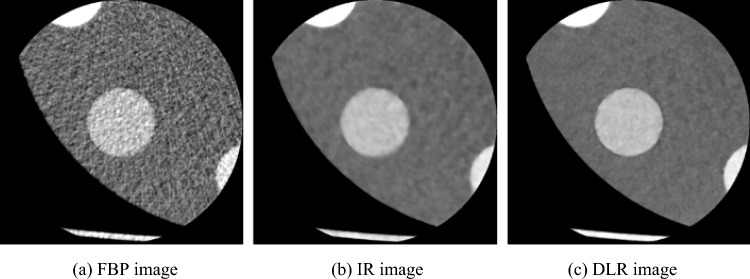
CT images of blood-equivalent module reconstructed using three noise reduction algorithms (Window level: 40 HU, Window width: 400 HU)
